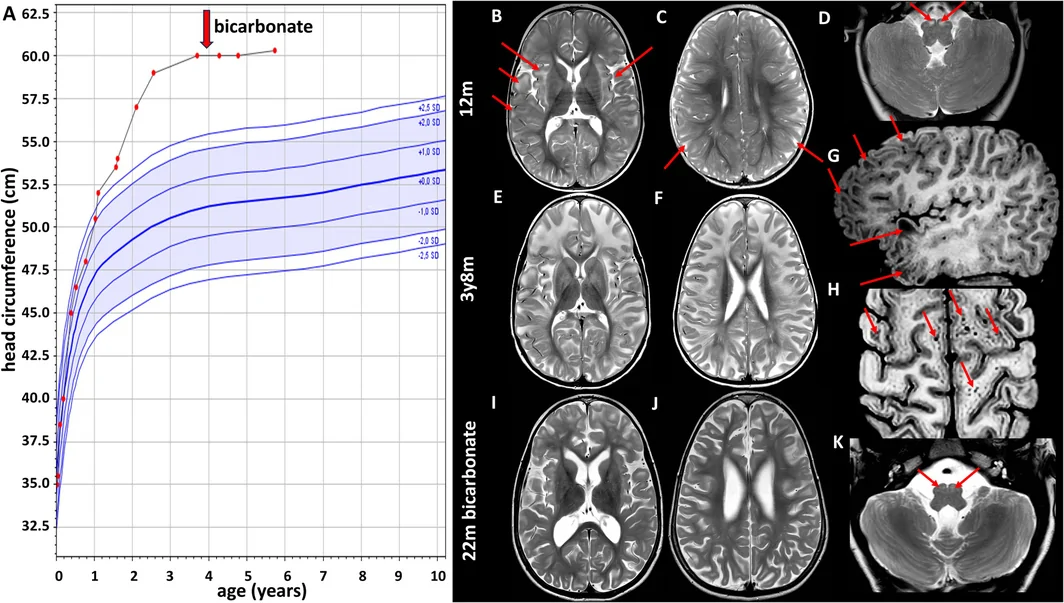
Brain MRI and head circumference in patient 2. (A) Head circumference. The red arrow indicates the start of bicarbonate treatment. (B–D) The first MRI at 12m shows minor abnormalities restricted to the directly subcortical cerebral white matter (arrows in B, C) and signal abnormalities in the pyramids and hilum of the inferior olives in the medulla (arrows in D). (E–H) Follow‐up MRI at 3y8m shows an increase in extensiveness and degree of swelling of the cerebral white matter abnormalities, with a decreasing zone of normal periventricular white matter (E, F). Especially, the directly subcortical white matter is rarefied to cystic (arrows in G; also note the anterior temporal cyst). A pattern of enlarged perivascular spaces is seen in the cerebral white matter (arrows in H). (I–K) MRI after 22 months treatment shows a marked decrease in the cerebral white matter edema (I, J) and resolution of the signal abnormalities in the medulla (arrows in K). y, years; m, months.
